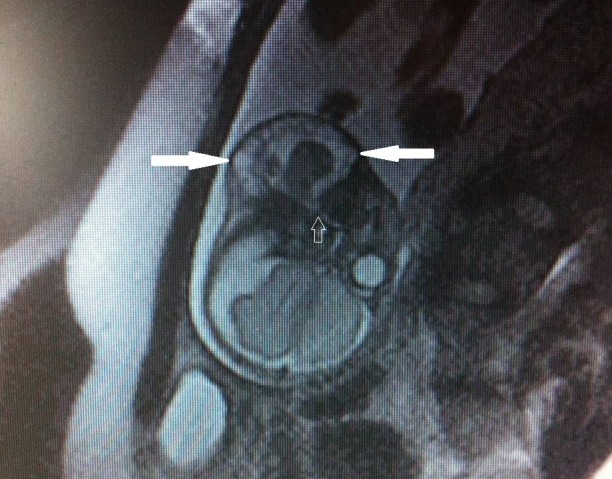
Figure 1: Fetal MRI showing a cervico-mandibular mass.
